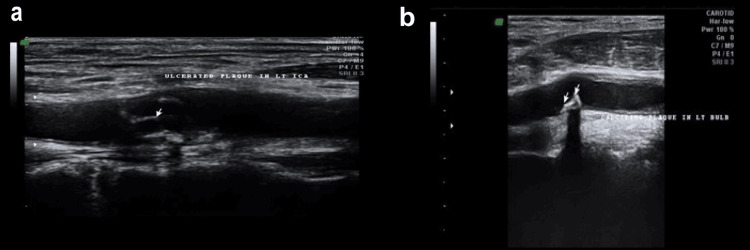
Ultrasonographic images showing ulcerated atherosclerotic plaques in the left carotid artery a: longitudinal B-mode ultrasonographic image demonstrating an ulcerated plaque in the left internal carotid artery (ICA), the plaque shows an irregular surface with a distinct ulcer crater (arrow), indicating plaque rupture; b: transverse sonographic image showing an ulcerated plaque in the left carotid bulb, the echolucent area within the plaque (arrows) represents the ulcer cavity with disrupted fibrous cap margins.
